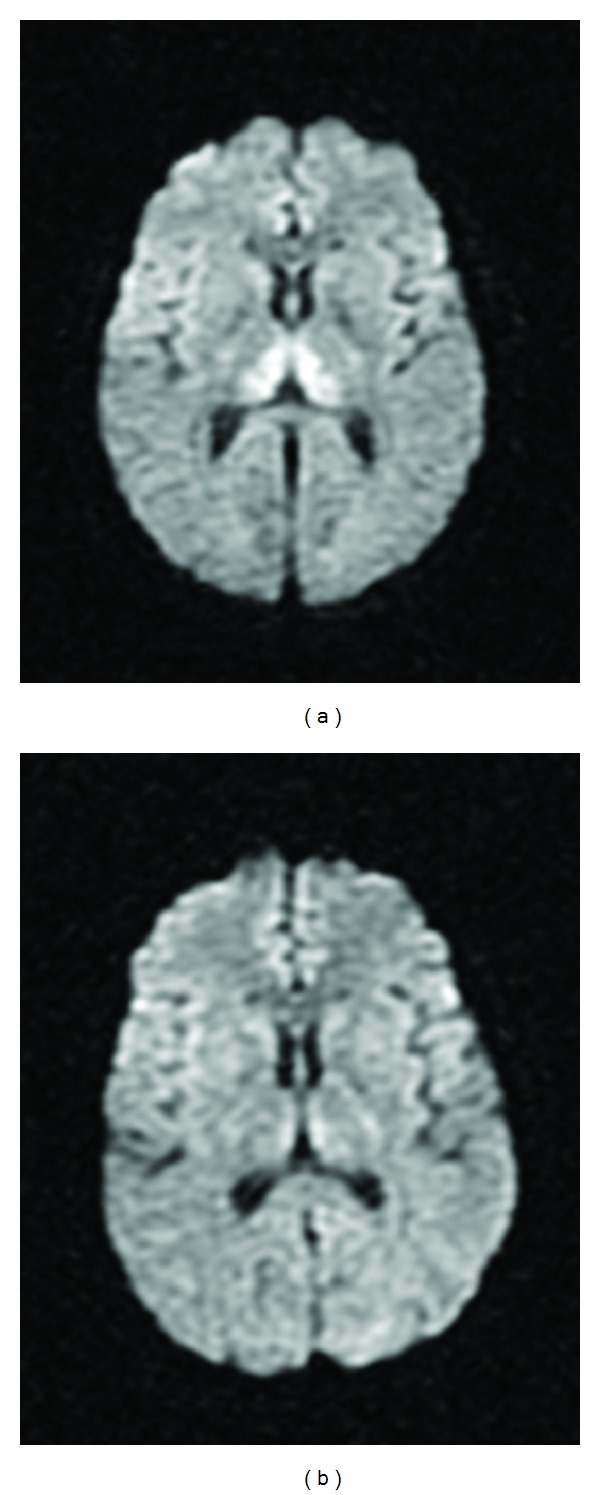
Corresponding DWI MRIs showing bilateral thalamic signal abnormalities one day (a) and seven days (b) after admission.
